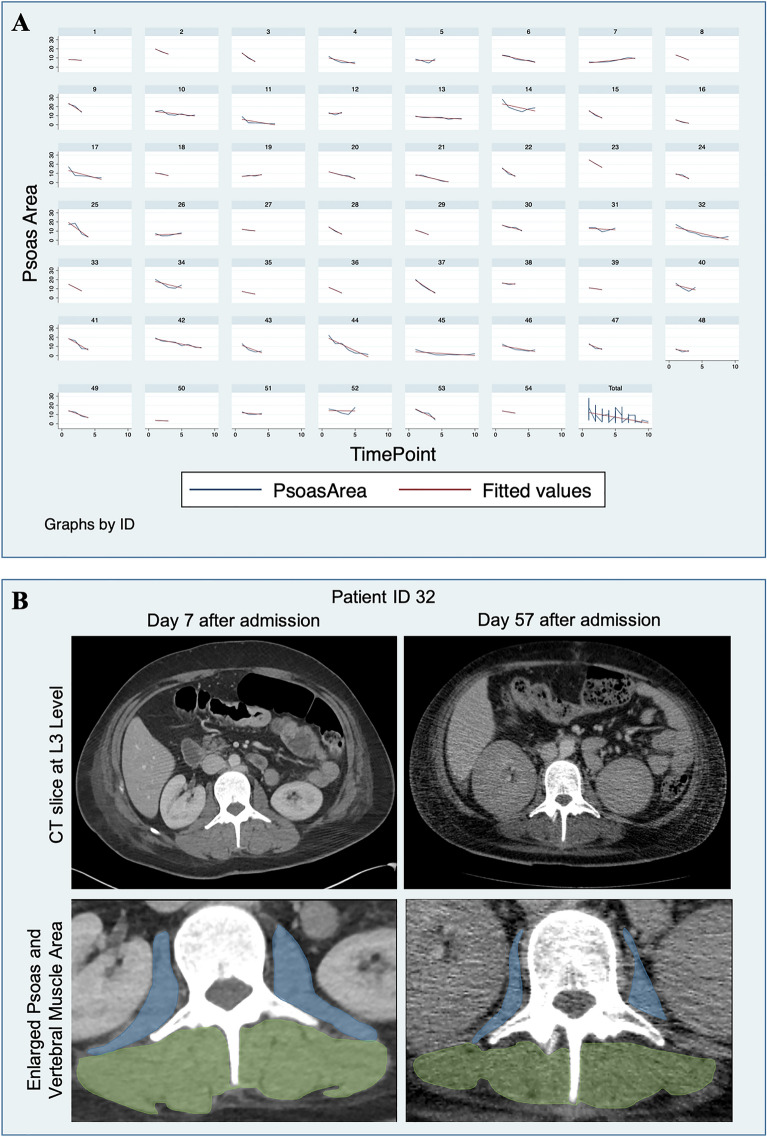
A Intermittent monitoring of psoas muscle area in 54 critically ill patients with ARDS due to SARS-CoV-2 infection. Metrics were collected at at least three time points using an AI-based segmentation tool applied to routinely performed CT scans. B Depicted are two CT slices used to perform body composition analysis at the level of lumbar vertebra 3 and the respective magnifications for better visualization of the psoas muscle. These example measurements, taken on days 7 and 57 after hospital admission, are from a young patient (ID 32) who survived the infection. Within the 50 days separating the scans, there is a distinct decrease in area of the psoas (blue) and the autochthonous back muscles (green)
